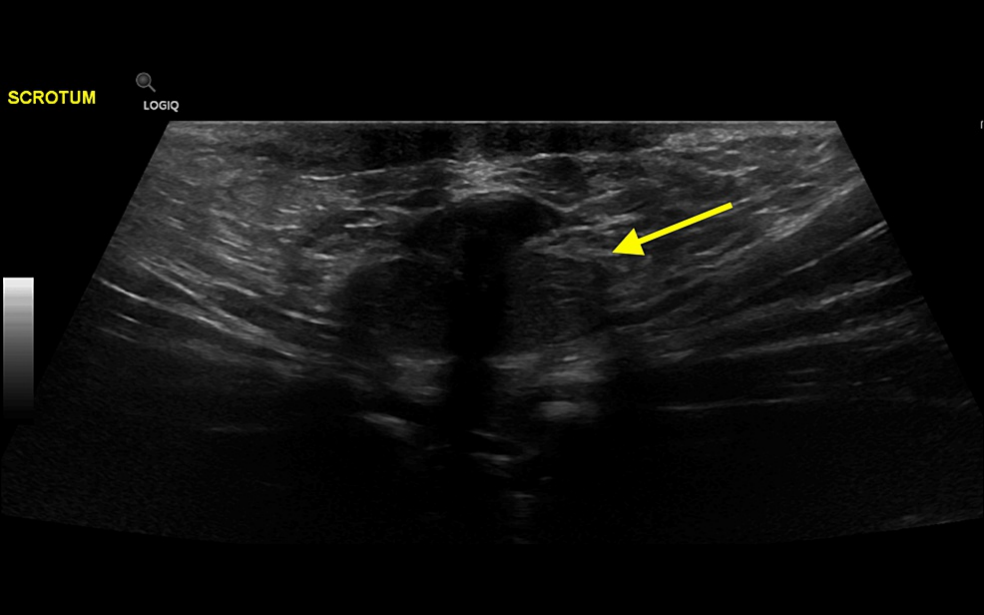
Ultrasound image of the scrotum shows empty scrotum (yellow arrow).
